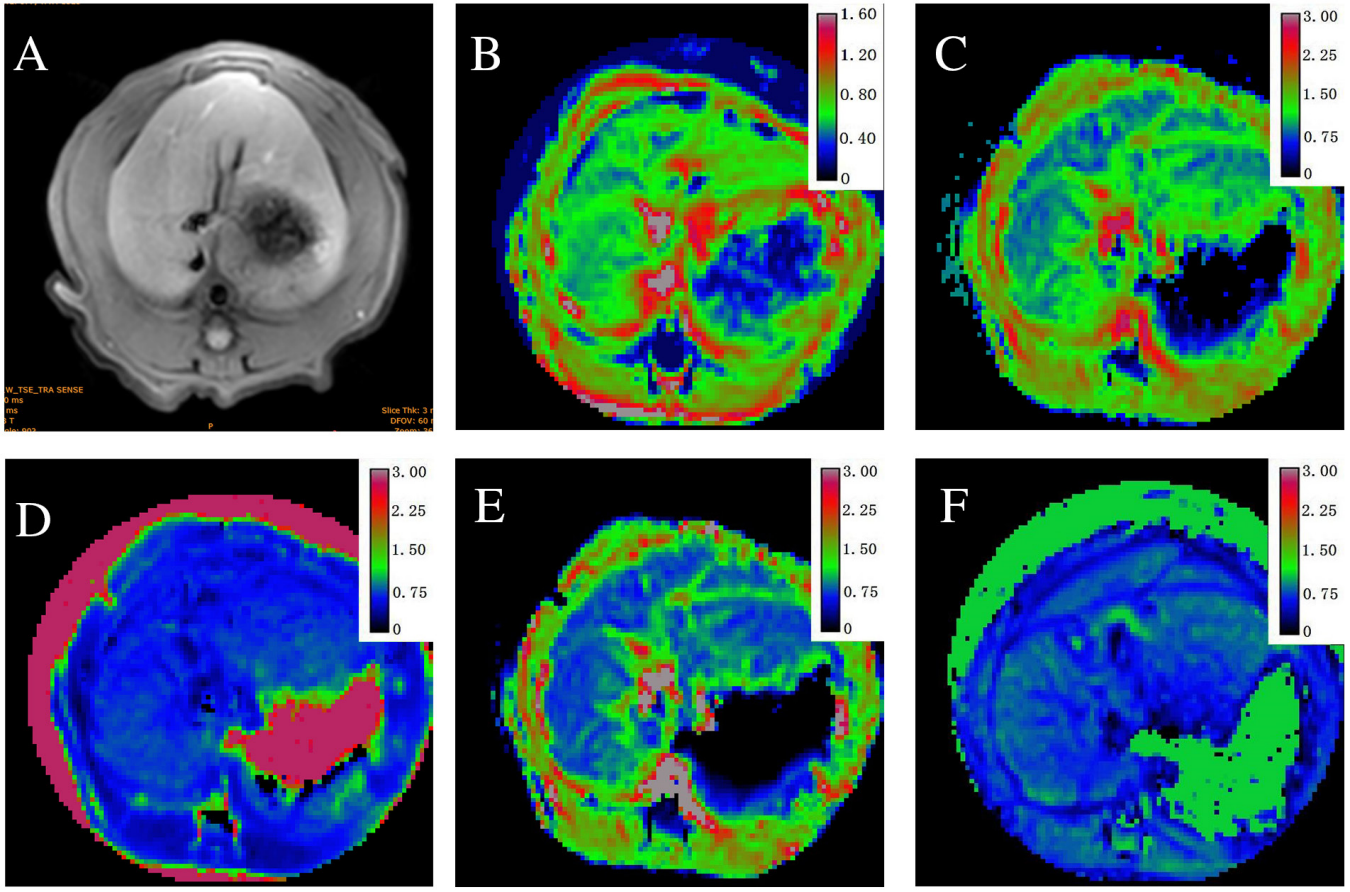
Axial MR images in sequence from a stage F2 fibrosis induced by carbon tetrachloride (CCl4) (A) T1-weighted image; (B) mean values (0.92×10^−3^ mm^2^/s) shown by a map of apparent diffusion coefficients (ADC); (C) mean values (1.10×10^−3^ mm^2^/s) shown by the D_app_ map; (D) mean values (1.02) shown by the K_app_ map; (E) mean values (0.76×10^−3^ mm^2^/s) shown by the DDC map; (F) mean values α (0.68) shown by a map.
